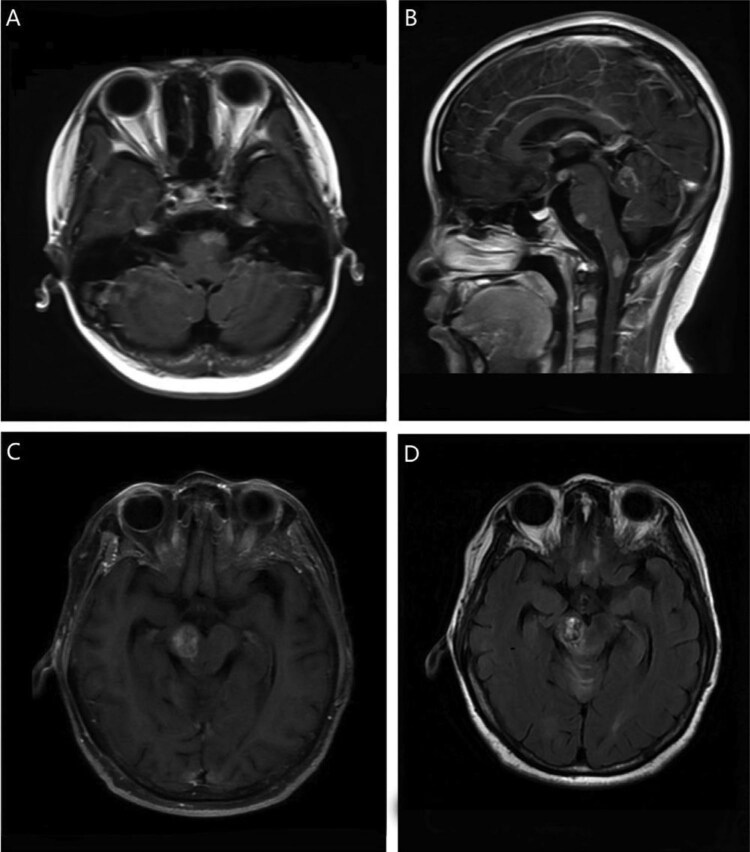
A 10-year-old female with primary brainstem lymphoma (PBSL). Axial (A) and sagittal (B) T1-postcontrast MR sequences showed multiple intense enhancing lesion in the medulla and pons. A 58-year-old female with PBSL. Axial T1-postcontrast MR sequence (C) showed an intense enhancing lesion in the midbrain. T2-FLAIR MR sequence (D) showed heterogeneous hyperintensity in the midbrain.
